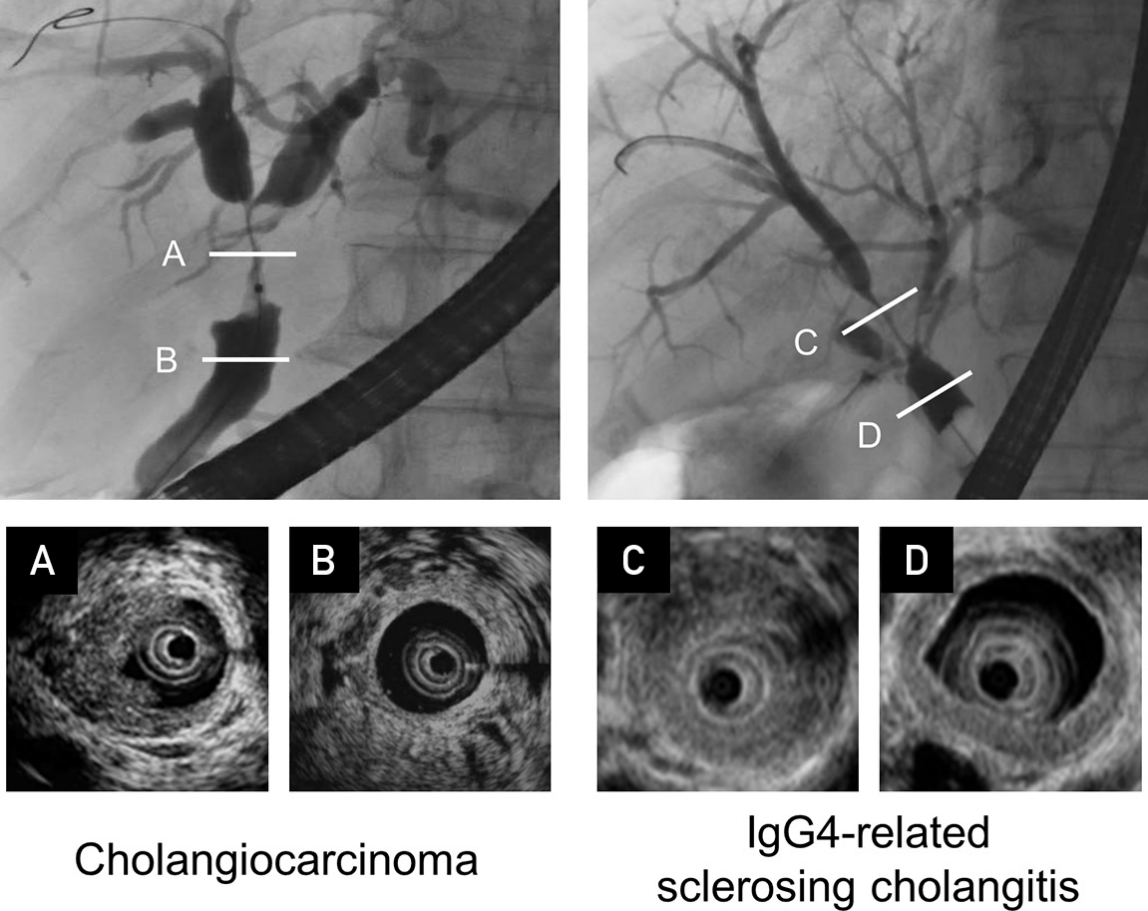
Intraductal ultrasound (IDUS) findings of strictures and nonstrictures of the bile duct enables differentiation of immunoglobulin G4 sclerosing cholangitis (IgG4-SC) from cholangiocarcinoma. There is asymmetrical thickening in strictures associated with cholangiocarcinoma (A), whereas there is symmetrical, concentric thickening observed in IgG4-SC (B). Similarly, there is symmetrical wall thickening in the nonstrictured segments of IgG4-SC (D), which is not present in cholangiocarcinoma (C); this is a specific finding for IgG4-SC (D).
